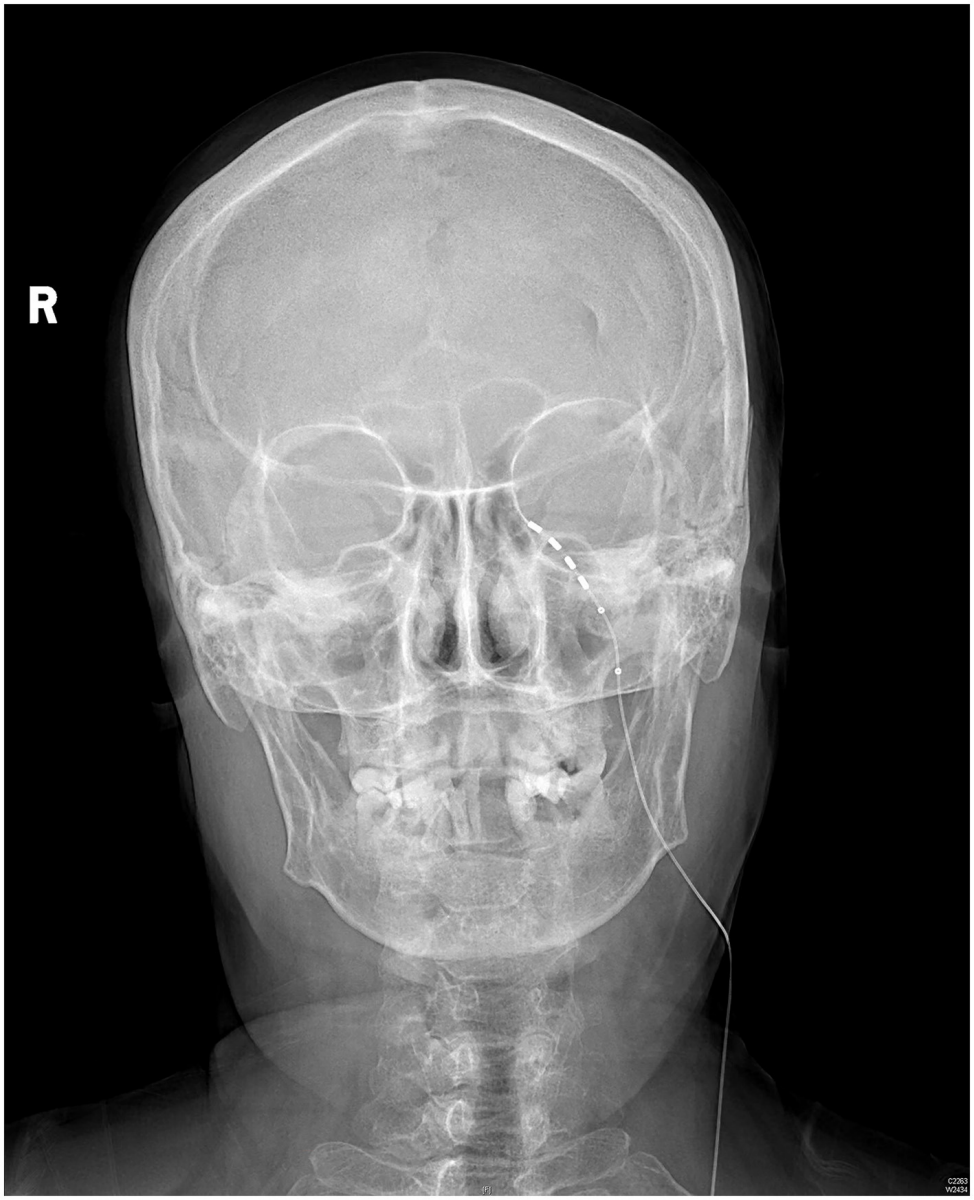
A skull-base x-ray of the anteroposterior view.
